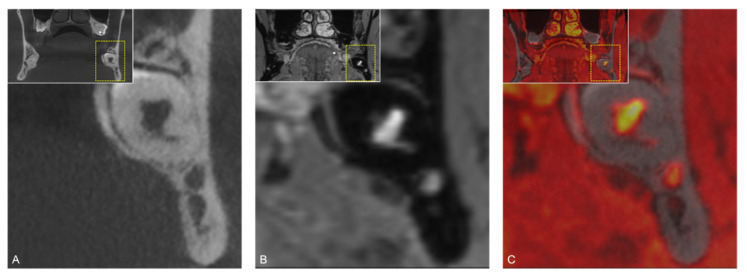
(A) CBCT, (B) MRI, and (C) fused CBCT/MRI scans of the positional relationship between the mandibular canal (MC)/inferior alveolar nerve (IAN) and the mandibular third molar (MTM). This borderline case visualizes the advantages of the selected imaging modality and their respective limitations. Additional relevant information, whether direct visualization of the IAN is possible or only the bony borders of the MC can be displayed, is presented depending on the imaging modality.
